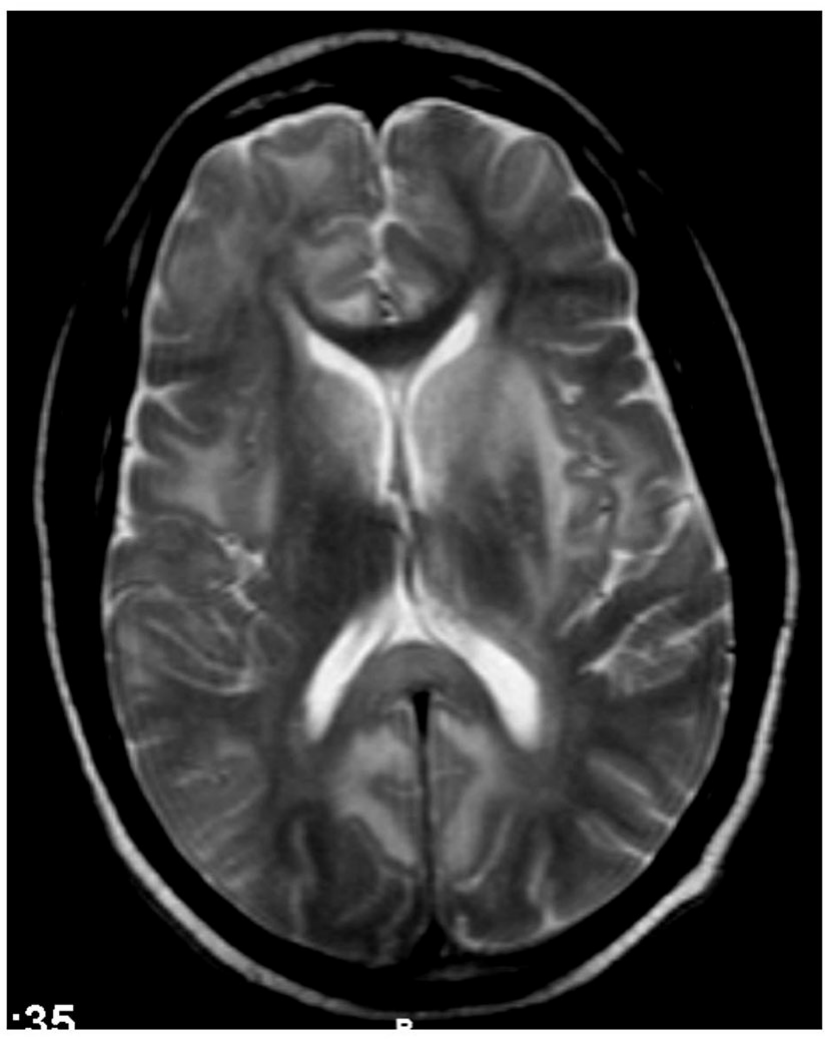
Case 12: Brain MR imaging (axial T2-weighted image) demonstrating multiple confluent white matter hyperintensities. There are significant hyperintensities bilaterally in the caudate, partly extending into the adjacent anterior putamen but sparing the thalami. The genu of corpus callosum is strikingly spared but the splenium/posterior body shows hyperintensities.
